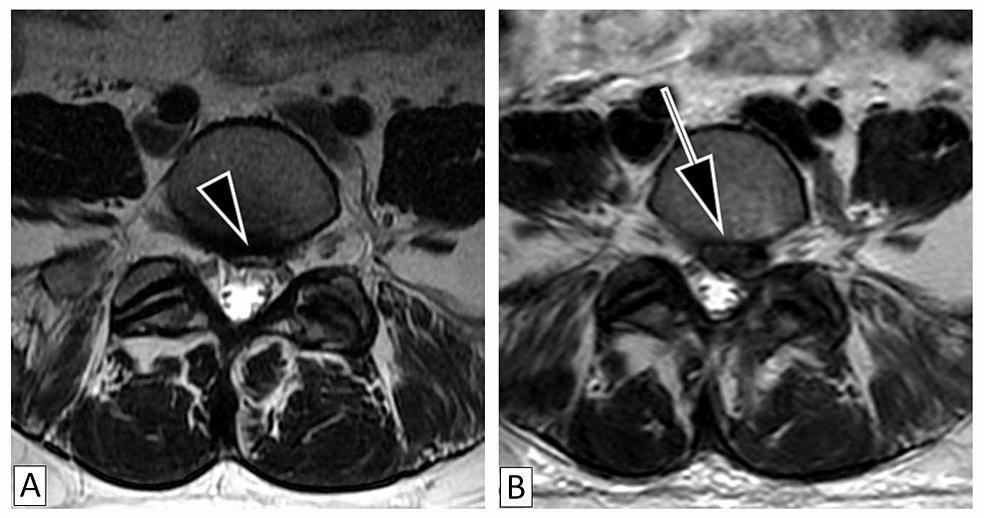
Magnetic Resonance Imaging of the High-Intensity Zone and Disc Extrusion Patient (Case 3) with magnetic resonance imaging revealing high-intensity zones (2A) indicated by the small arrowhead correlating to areas of disc extrusion (2B) indicated by the arrow.
